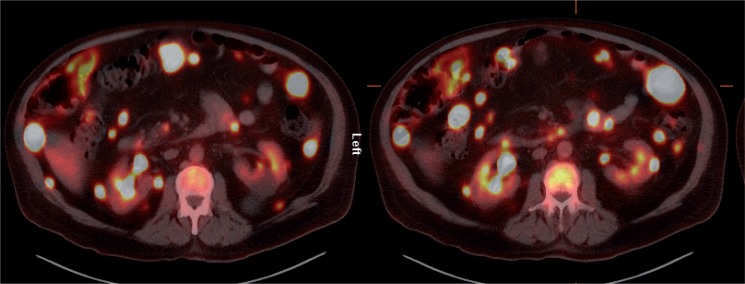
Axial ^18^F-FDG PET/CT fusion images. Foci of markedly increased ^18^F-FDG uptake are shown in the peritoneal cavity, and in exophytic lesions in the kidneys bilaterally.
